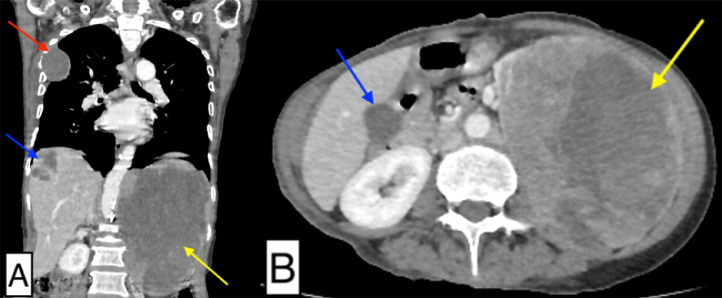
Contrast enhanced computed tomography (CT) of thorax and abdomen. (A) Coronal and (B) Axial images showing a large heterogeneously enhancing soft tissue mass lesion measuring 16 × 13.1 × 12.1 cm arising from the upper pole of the left kidney (yellow arrow). Well circumscribed lytic lesion measuring 6.6 × 4.5 × 4.7 cm arising from the lateral aspect of right 4th rib (red arrow). Multiple enhancing mass lesions in the liver parenchyma (blue arrow).
